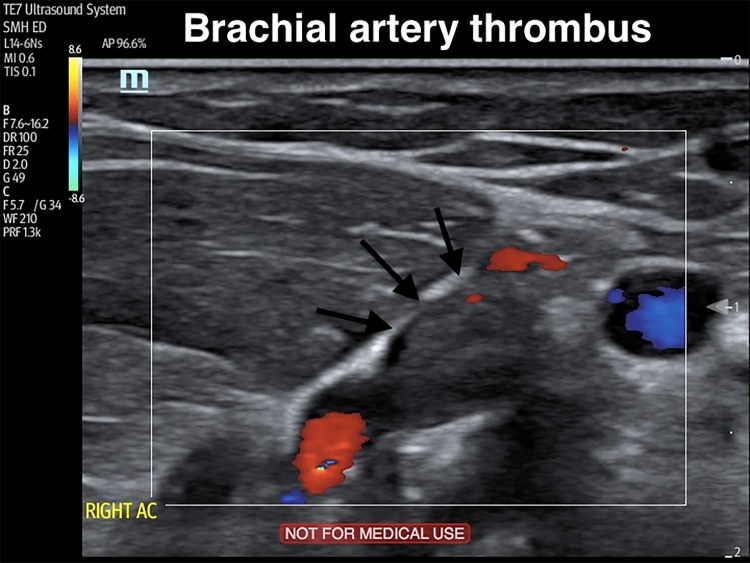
Still image of a duplex ultrasound demonstrating a brachial artery thrombus (arrows).
